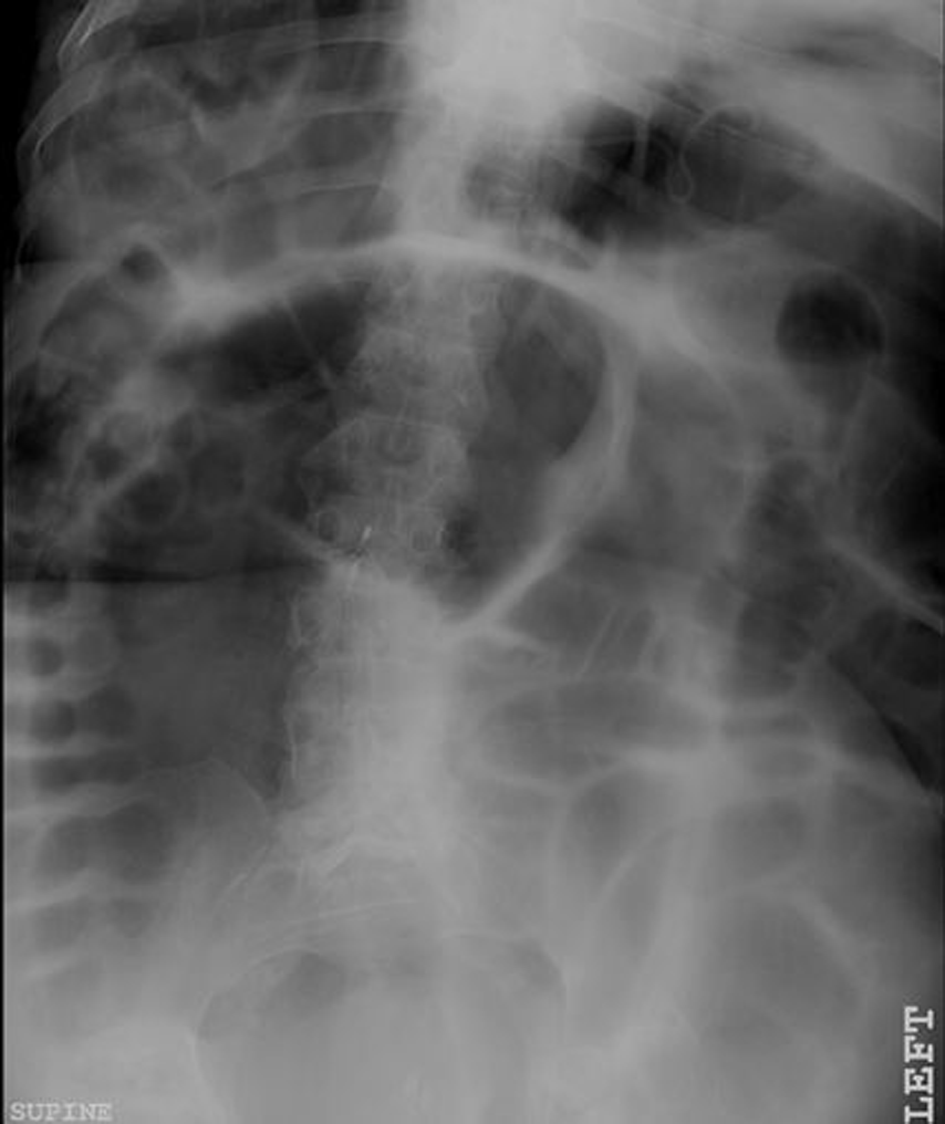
Plain X-Ray abdomen, supine position demonstrating volvulus without fluid levels.
